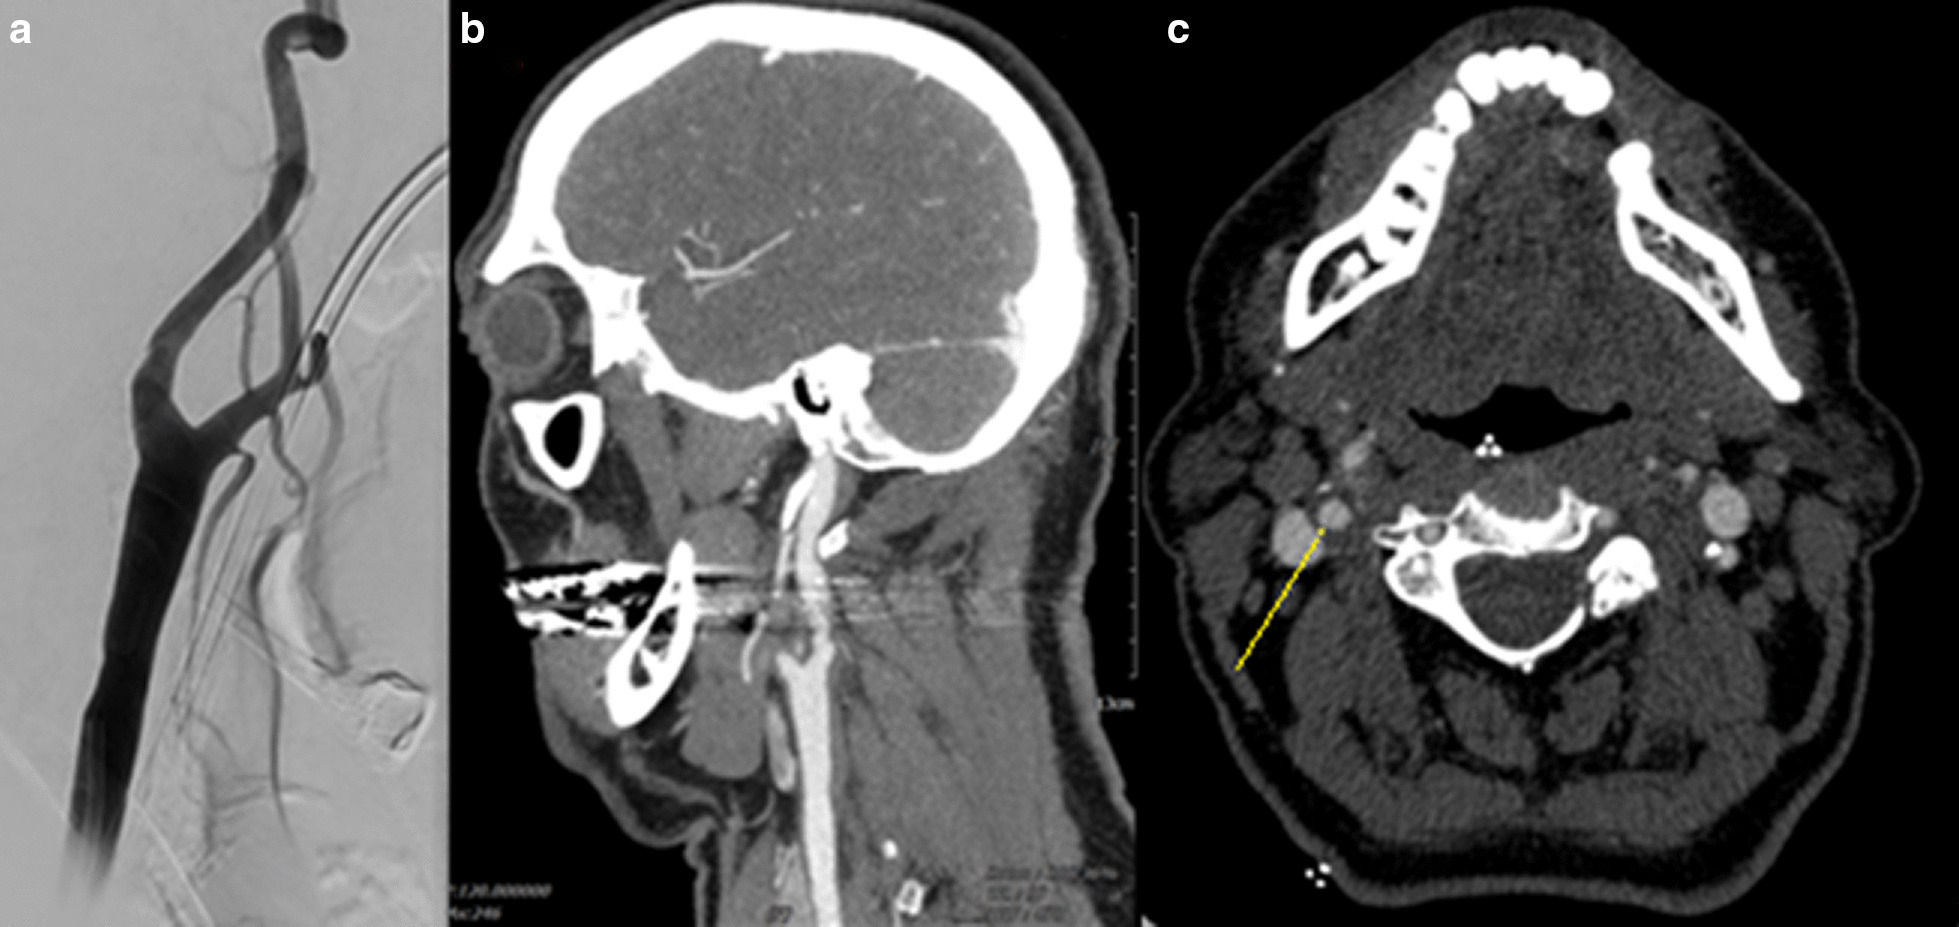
a Digital subtraction angiography of the right internal carotid artery. b Sagittal view of mild internal carotid artery stenosis. c Axial view of the CT-angiogram, the yellow bar shows a carotid web in the right internal carotid artery. With permission of Prof. Ringelstein, Department of Radiology and Neuroradiology, Kliniken Maria Hilf
